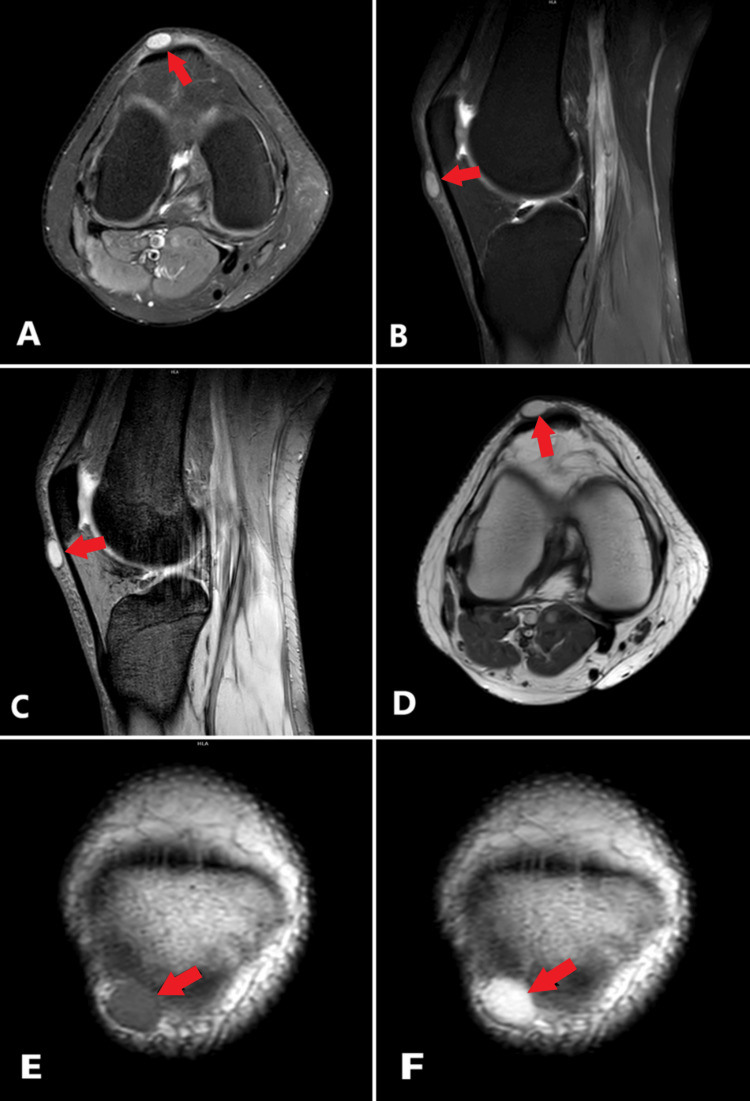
In the contrast-enhanced MRI examination of the right knee, the axial (A) and sagittal (B) proton density (PD)-weighted images show a well-defined hyperintense nodular lesion with capsular formation in the subcutaneous prepatellar area adjacent to the anteroinferior aspect of the patella and patellar tendon (red arrows). A similar hyperintense appearance was noted on multi-echo fast field echo (MFFE) T2-weighted images (C, red arrow). On coronal pre-contrast T1-weighted images (E), the lesion appeared homogenous and iso-hyperintense to muscle (red arrow). Post-contrast axial (D) and coronal T1-weighted images (F) demonstrated diffuse and homogeneous gadolinium enhancement of the lesion (red arrows).
